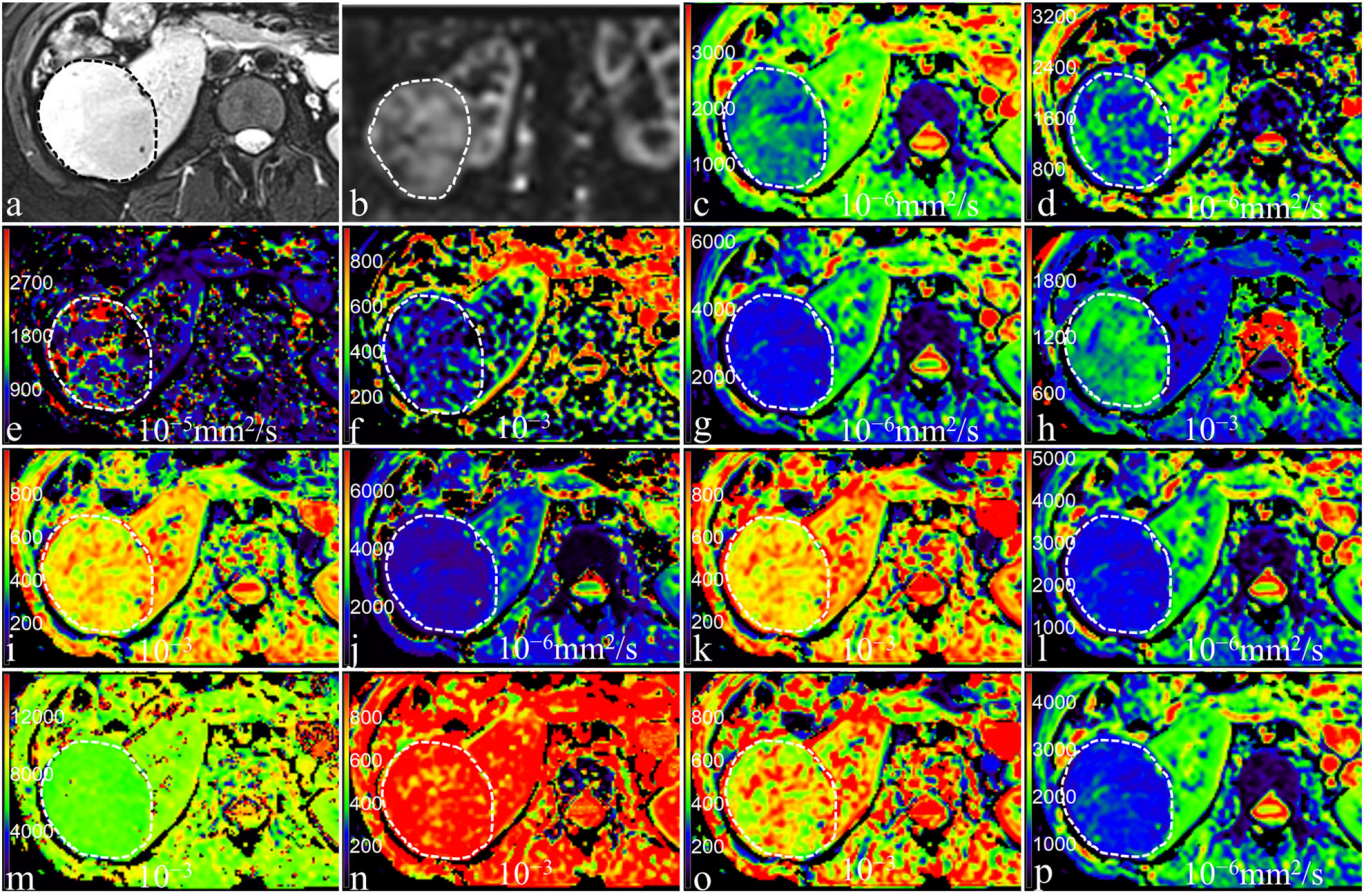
A 43-year-old woman with pathologically confirmed chromophobe renal cell carcinoma in the right kidney. a Axial T2-weighted imaging shows a moderate-signal-intensity mass; b RBF map shows the perfusion level of mass, with the RBFmean and RBFpeak of 156.13 mL/100 g/min and 272.00 mL/100 g/min; c–p pseudo-colorized images showed the Mono_ADC (c), IVIM_D (d), IVIM_D* (e), IVIM_f (f), DKI_MD (g), DKI_MK (h), SEM_α (i), SEM_DDC (j), FROC_β (k), FROC_D (l), FROC_μ (m), CTRW_α (n), CTRW_β (o), and CTRW_D (p), with the values of 1.15 × 10^−3^ mm^2^/s, 0.93 × 10^−3^ mm^2^/s, 11.82 × 10^−3^ mm^2^/s, 15.38%, 1.43 × 10^−3^ mm^2^/s, 0.85 × 10^−3^, 0.69 × 10^−3^, 1.17 × 10^−3^ mm^2^/s, 0.70 × 10^−3^, 0.85 × 10^−3^ mm^2^/s, 3.82 × 10^−3^, 0.93 × 10^−3^, 0.71 × 10^−3^, and 1.18 × 10^−3^ mm^2^/s, respectively
